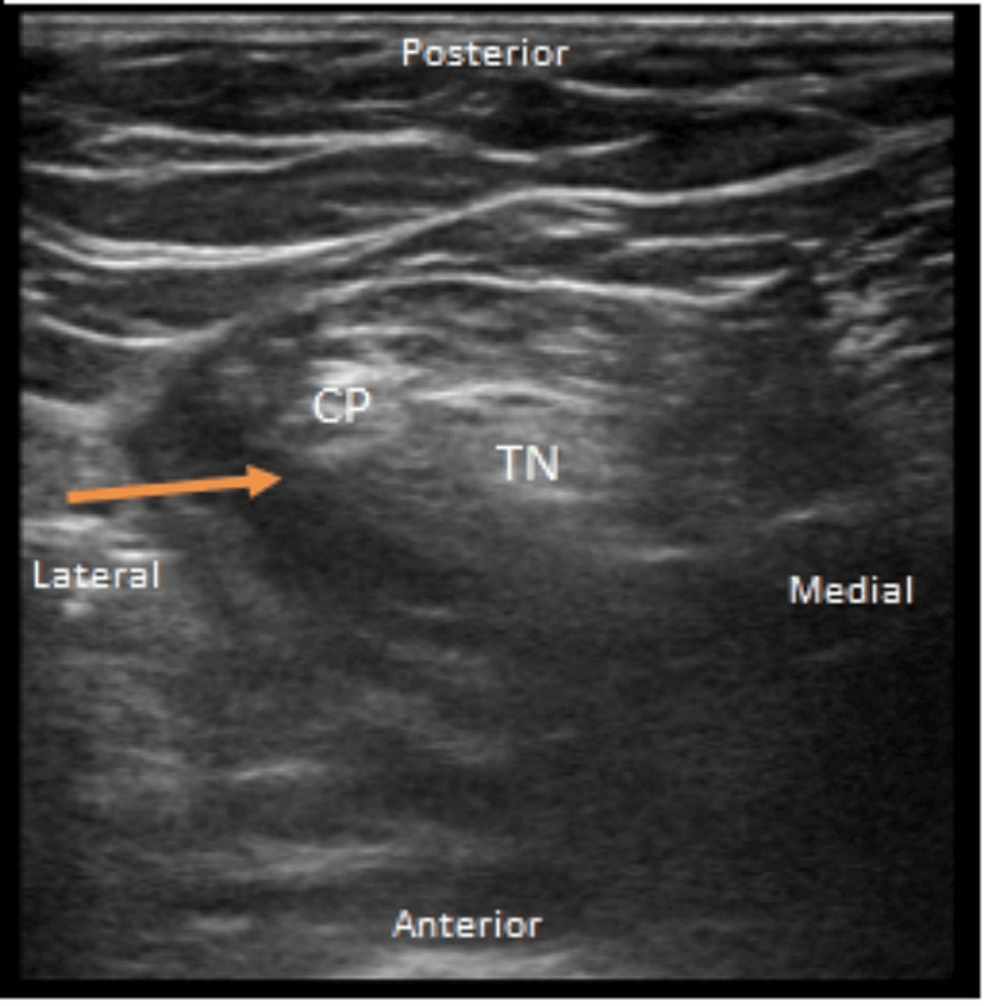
Ultrasound-guided right popliteal catheter placement with linear probe The catheter was at a skin depth of 6 cm and the needle insertion depth was 6 cm. Tuohy 18 g 10 cm needles with 20 g catheters and injection assessment showed negative aspiration, and no paresthesia on injection. Orange arrow: the in-plane tip of the needle CP: common peroneal; TN: tibial nerve
